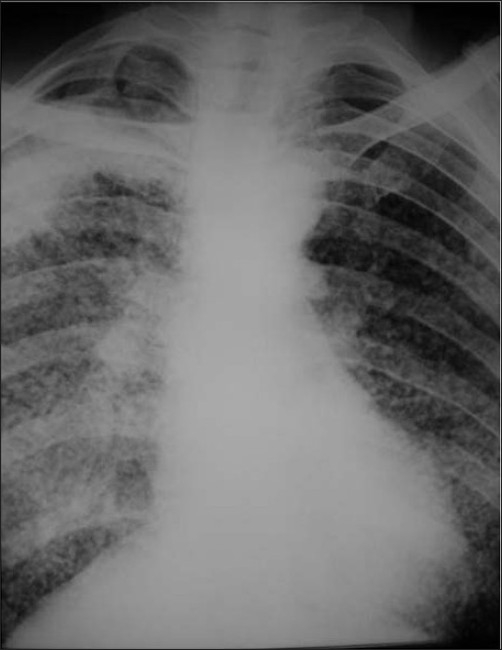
A chest radiograph shows multiple high density nodules due to a lifetime exposure to hematite and silica
